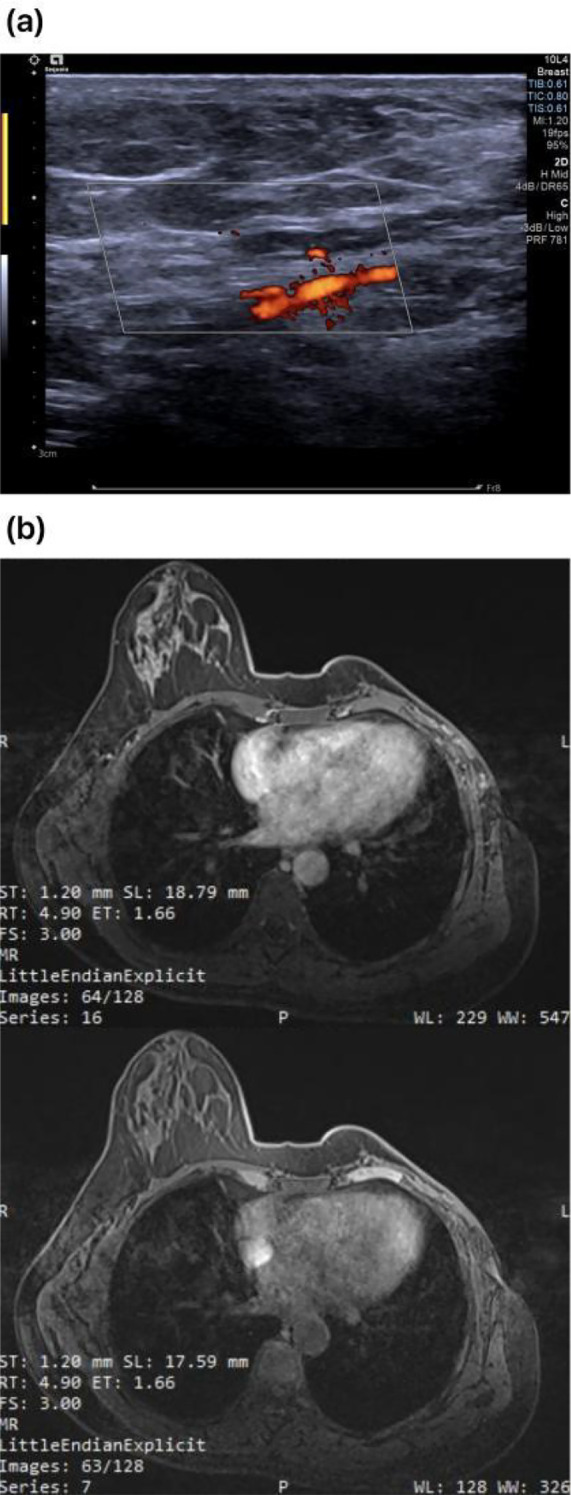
Follow-up images of breast color Doppler ultrasound (A) and breast MRI (B).
